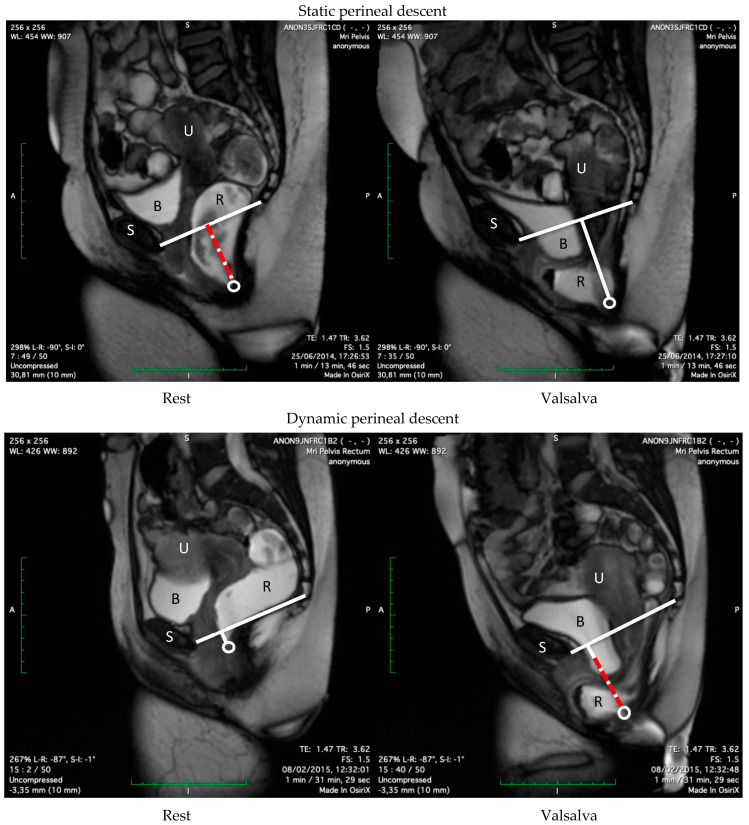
Perineal descent on MRI: *Static* perineal descent (red dashed) i.e., large difference between position of the ARJ and the PCL at rest, with subsequent minimal difference in movement of the ARJ during maximum Valsalva. *Dynamic* perineal descent (red dashed) i.e., large difference in movement of the ARJ during maximum Valsalva, following a minimal difference between position of the ARJ and the PCL at rest. S = symphysis pubis, B = bladder, R = rectum, U = uterus, circle = ARJ.
